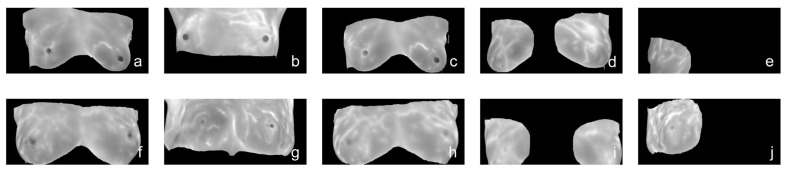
Preprocessing of normal breast samples and breast cancer MRI images, including the image of normal breast samples (a), image rotated 180 degrees (b), horizontal mirror image (c), cropped original image (d), and lesion image (e); image of breast cancer samples (f), image rotated 180 degrees (g), horizontal mirror image (h), cropped original image (i), and lesion image (j).
